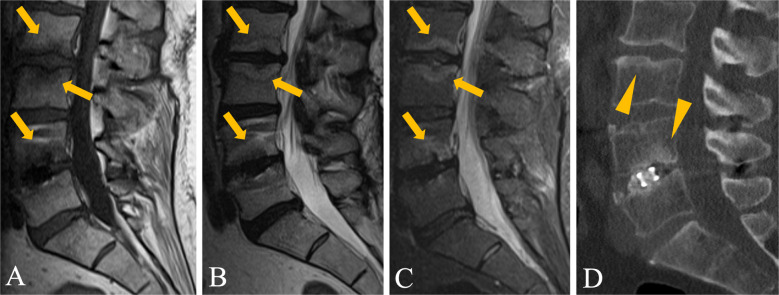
Sagittal T1-weighted images (a) and T2-weighted images (b) of a 57-year-old female patient, who underwent L4/5 interbody fusion and cage implantation. Arrows indicate the Modic type 1 changes of the endplates, along with bone marrow edema-like lesions in the fluid-sensitive short tau inversion recovery image (c). The subsequent photon-counting detector CT examination (d) revealed minor sclerosis along the vertebral endplates of L3 and L4 (arrowheads). The LASSO model estimated probabilities of MC-1 changes of 0.92 at L2, 0.90 at L3, 0.85 at L4, 0.24 at L5, and 0.05 at S1
